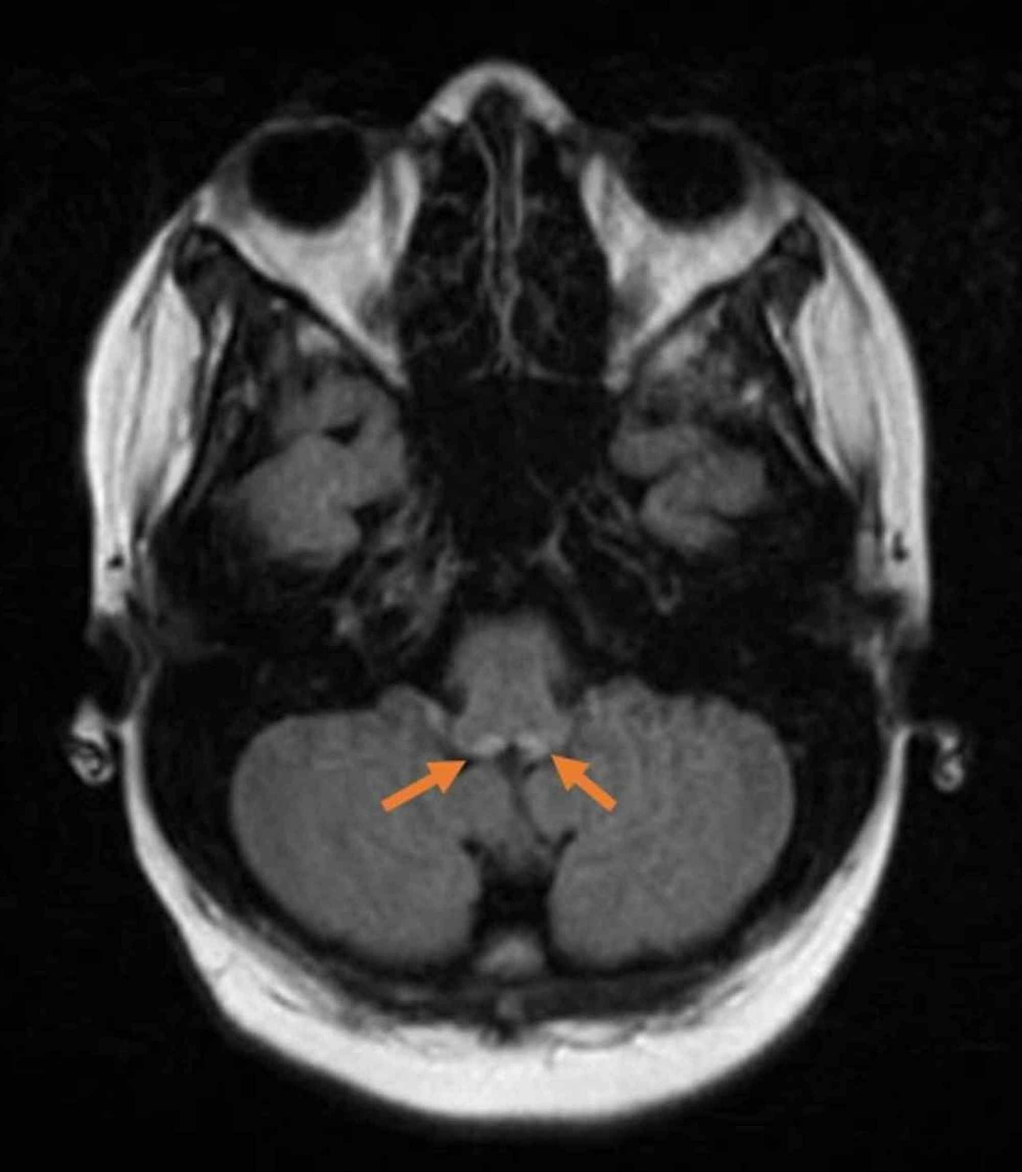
MRI brain non-contrast showing T2 signal abnormality in medial thalami bilaterally Equivocal signal abnormality in periaqueductal gray nuclei and increased signal present within medial vestibular nuclei in the dorsal medulla as seen in Wernicke's encephalopathy.
